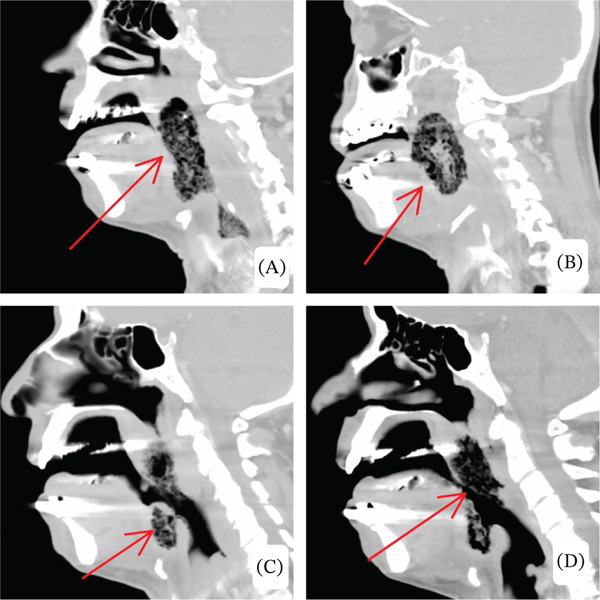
(A–D) Sagittal contrast‐enhanced CT images at multiple levels demonstrate diffuse tonsillar enlargement with central and submucosal gas locules, associated with extensive surrounding inflammatory changes, representing the same emphysematous tonsillar infection seen on axial images. (C) At the periepiglottic level, there is circumferential inflammatory involvement with gas locules, resulting in marked airway narrowing.
